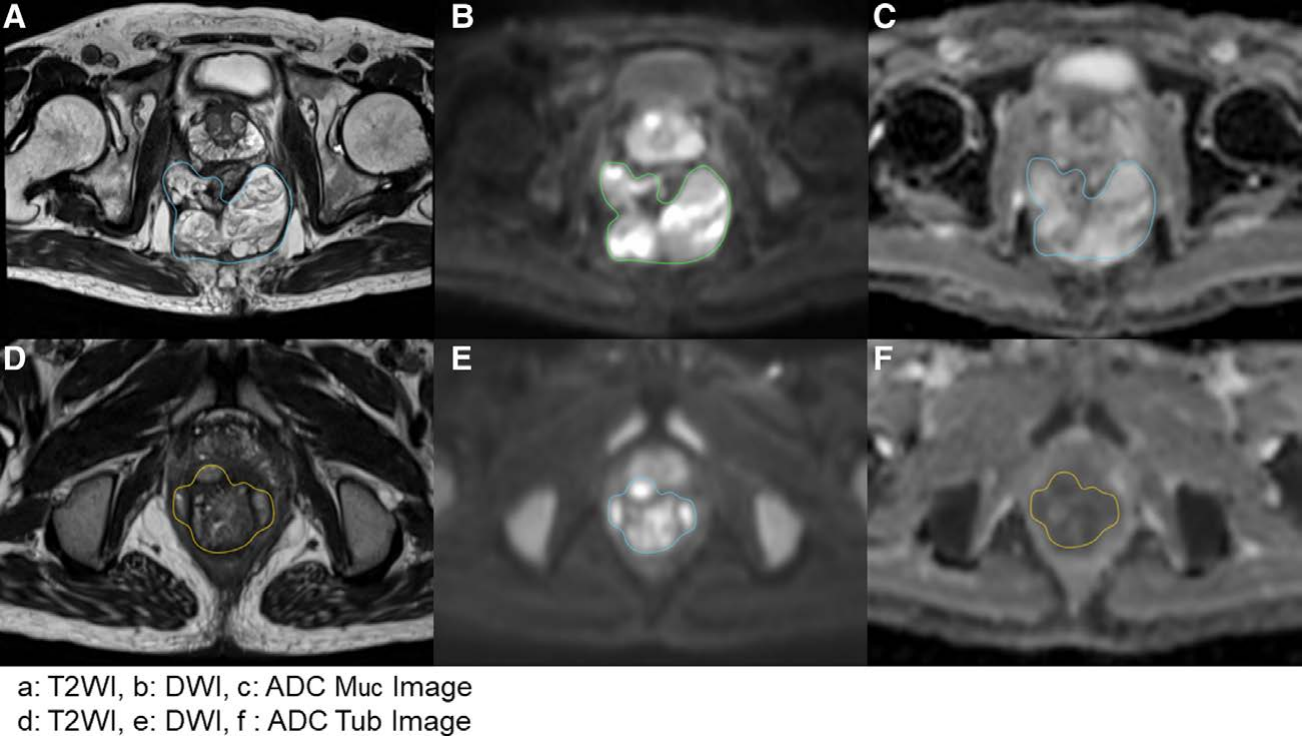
MRI images. (a–c) T2WI, DWI, and ADC images of a mucinous adenocarcinoma; (d–f) T2WI, and DWI, and ADC images of a tubular adenocarcinoma. ADC = apparent diffusion coefficient, DWI = diffusion-weighted imaging, MRI = magnetic resonance imaging, T2WI = T2-weighted imaging.
